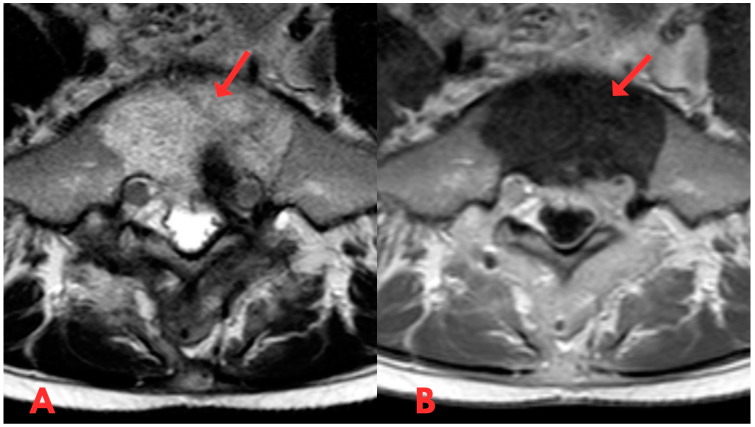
(A,B) Second T1- and T2-weighted axial MRI of the lumbar spine (T2W_TSE, T1W_TSE ax) showing an S1 vertebral lesion with a hyperintense signal on T2 (A) and hypointense on T1 (B) after a biopsy. Dynamics without change in the prevertebral and spinal canal.
